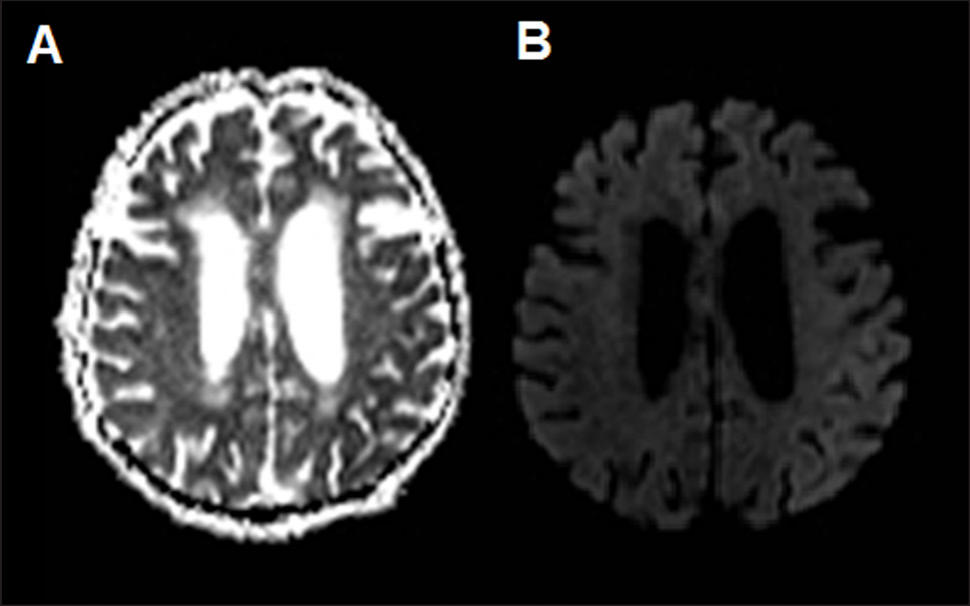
ADC (a) and b1000 DWI (b) show subtle diffusion restriction in the cortex of the left parietotemporal lobe, corresponding with the hyperperfused region observed on perfusion-CT.
